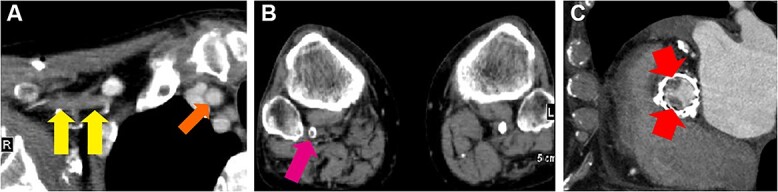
Contrast-enhanced computed tomographic images. Reduced contrast enhancement of the occluded right subclavian artery (A, two arrows on the left side) compared with that of the right common carotid artery (A, arrow on the right side), filling defect of the right popliteal artery (B, arrow) and transcatheter heart valve thrombosis (C, arrows) were detected.
